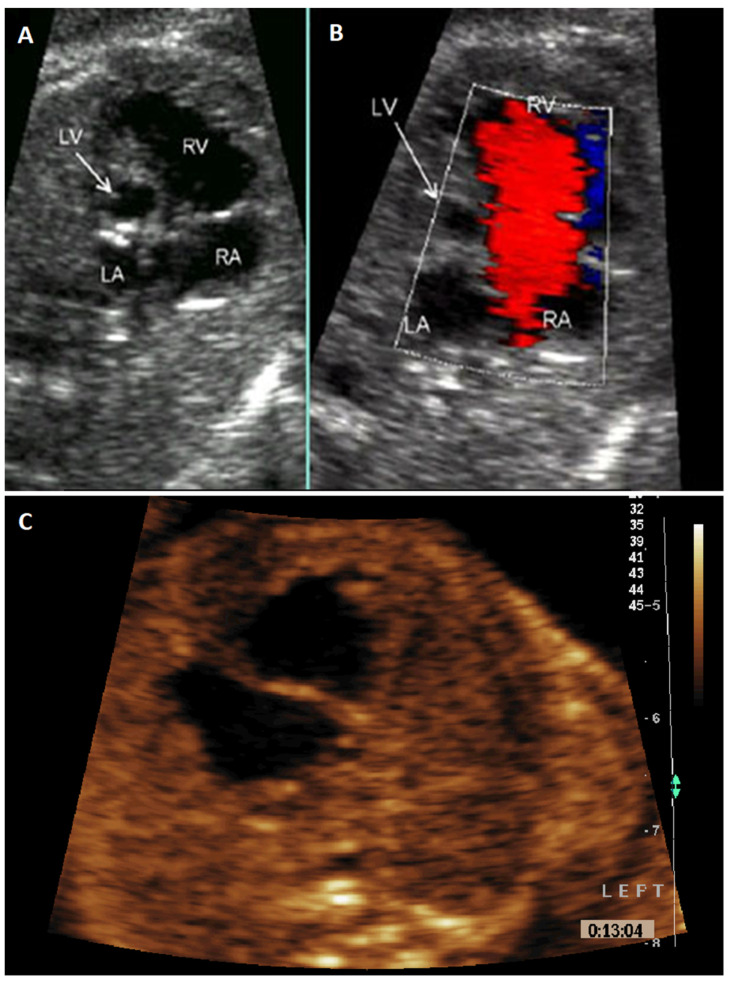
(A) Hypoplastic left heart syndrome fetal echocardiogram in a four-chamber view with noticeably reduced dimensions of the left ventricle compared to the right ventricle. (B) The same heart presented in color Doppler echocardiography. The embryogenesis of this heart is usually at 6–8th week after conception or even later on. (C) Single fetal echocardiogram. This heart in terms of embryology was earlier developed comparing with hypoplastic left heart syndrome, usually 4–5th week after conception. Legend: LA—left atrium, LV—left ventricle; RA—right atrium, RV—right ventricle.
